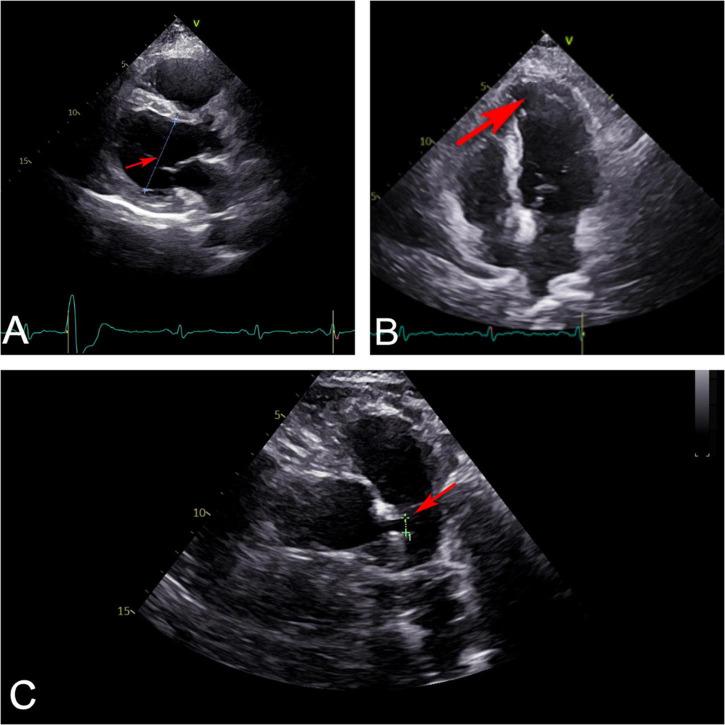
Ultrasonic cardiography showed that (A) left ventricle (red arrow) slightly enlarged from the parasternal long axis section view. (B) Apex of left ventricle (red arrow) bulged slightly outward from four-chamber view. (C) Left main coronary artery (red arrow) widened from random view.
